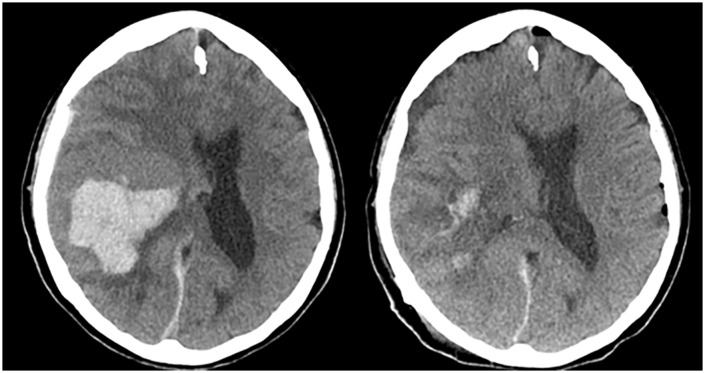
Examples of pre- and post-operative images after Aurora Surgiscope-based treatment of various intracranial pathologies. Non-contrast axial CT scans demonstrating evacuation of an intraparenchymal hematoma.
